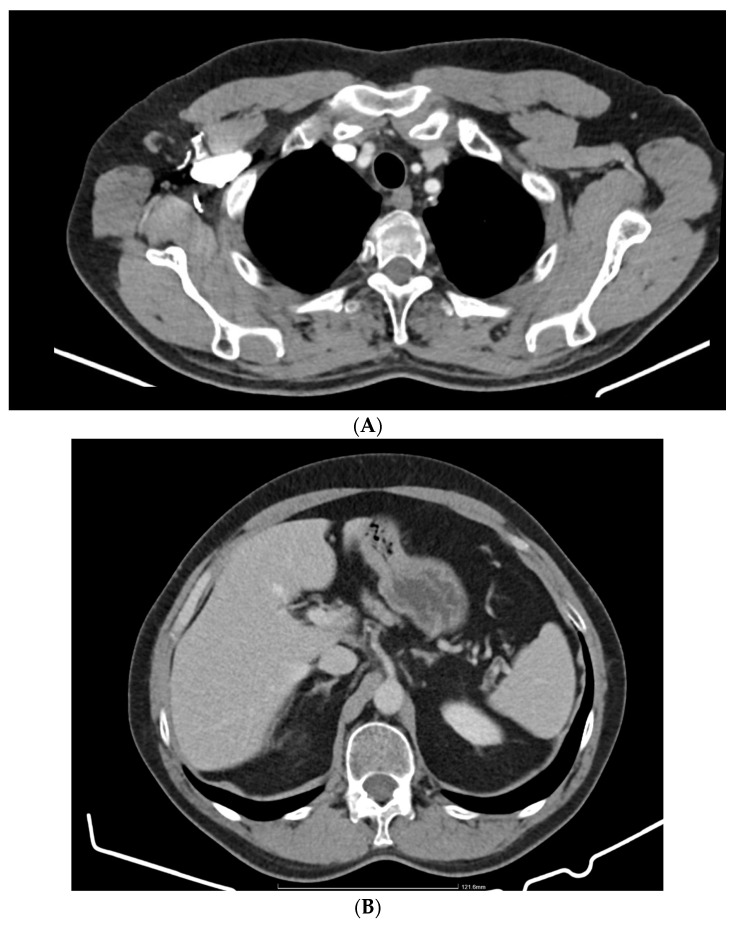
(A) March 2024 chest CT: normal appearance. (B) CT abdomen March 2024: normal appearance.
